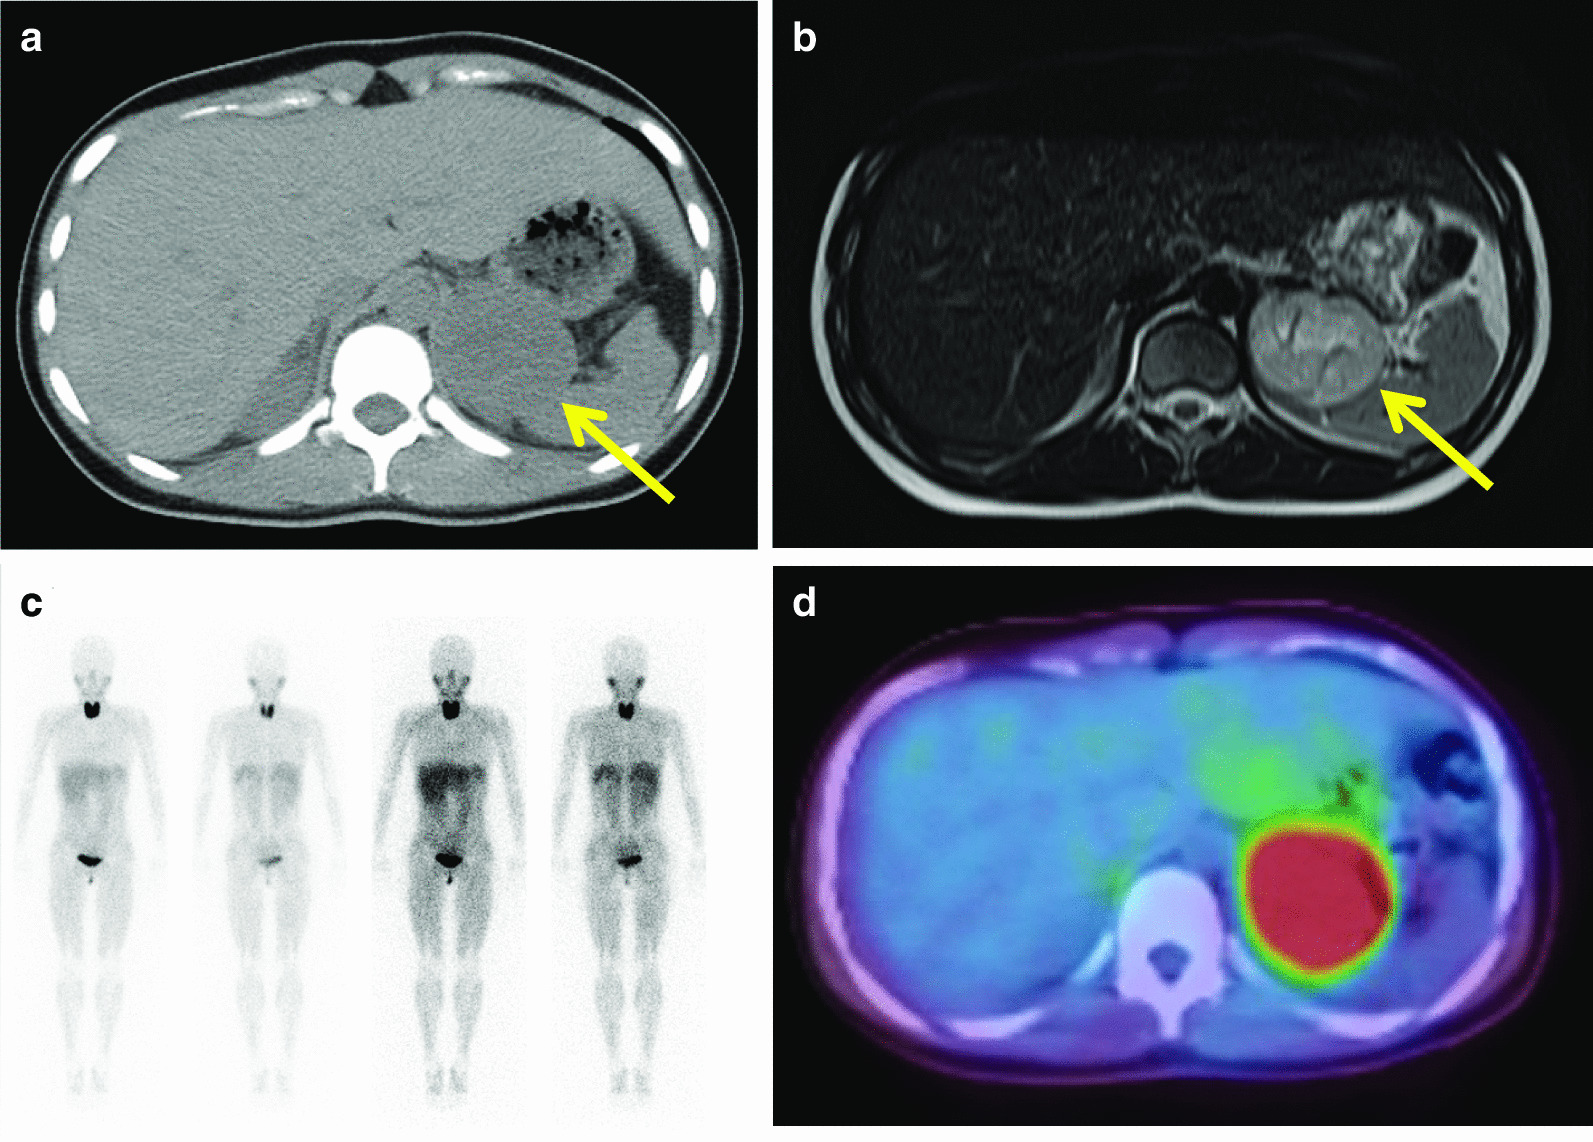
Abdominal computed tomography scan showing a 50 mm mass above the left kidney (arrow) (a). Abdominal MRI showing the mass is nonhomogeneous and of moderate intensity on T2-weighted images (arrow) (b). ^123^I-MIBG scintigraphy showing no accumulation in the mass (c). FDG-PET showing accumulation (SUVmax 10.9) in the mass (d). *CT*, computerized tomography; *FDG-PET*, fluorodeoxyglucose-positron emission tomography; *MIBG*, metaiodobenzylguanidine; *MRI*, magnetic resonance imaging; *SUVmax*, maximum standardized uptake value
